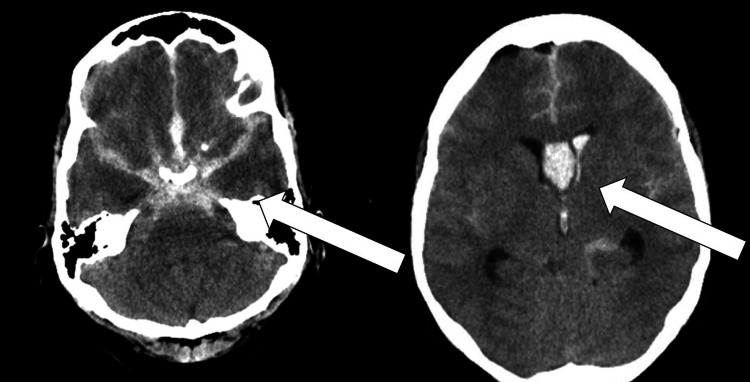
Initial CT imaging of the identified diffuse subarachnoid hemorrhage (left) and intraventricular extension (right). CT: computed tomography.
